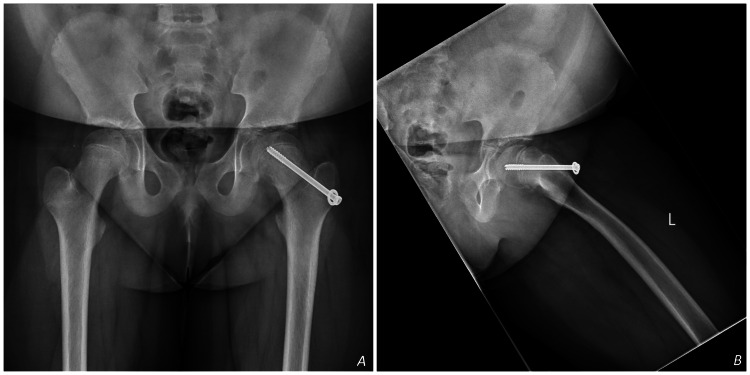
Post-operative X-rays, AP view of the pelvis (A) and Dunn view (B) of the left hip, showing percutaneous pin fixation of SUFE in the left hip. SUFE: Slipped Upper Femoral Epiphysis; AP: Anteroposterior
